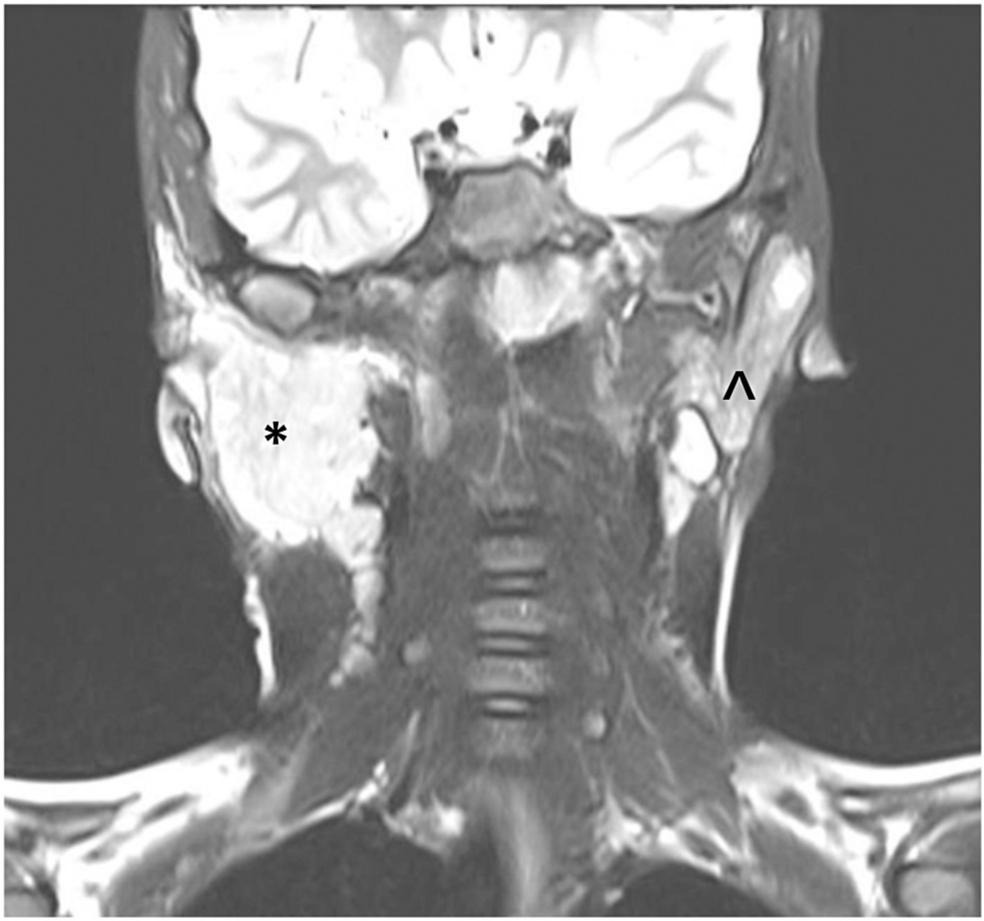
MRI of the patient reviling a right acute-on-chronic parotiditis (*) and chronic left parotiditis with mild atrophic changes (^)
